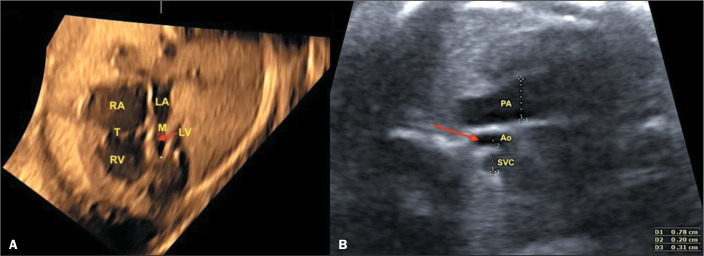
Fetal echocardiographic finding in a case of hypoplastic left heart
